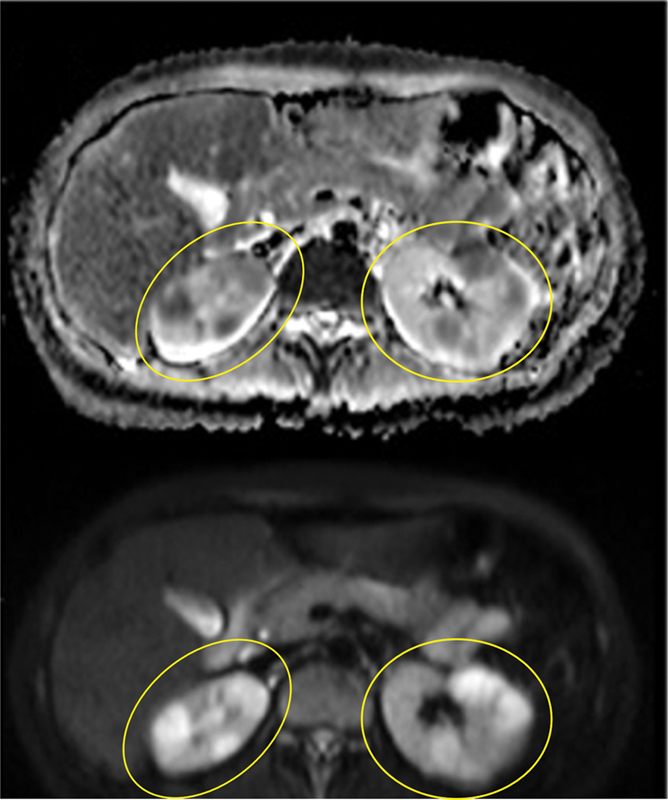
Abdominal magnetic resonance imaging with apparent diffusion coefficient map (
